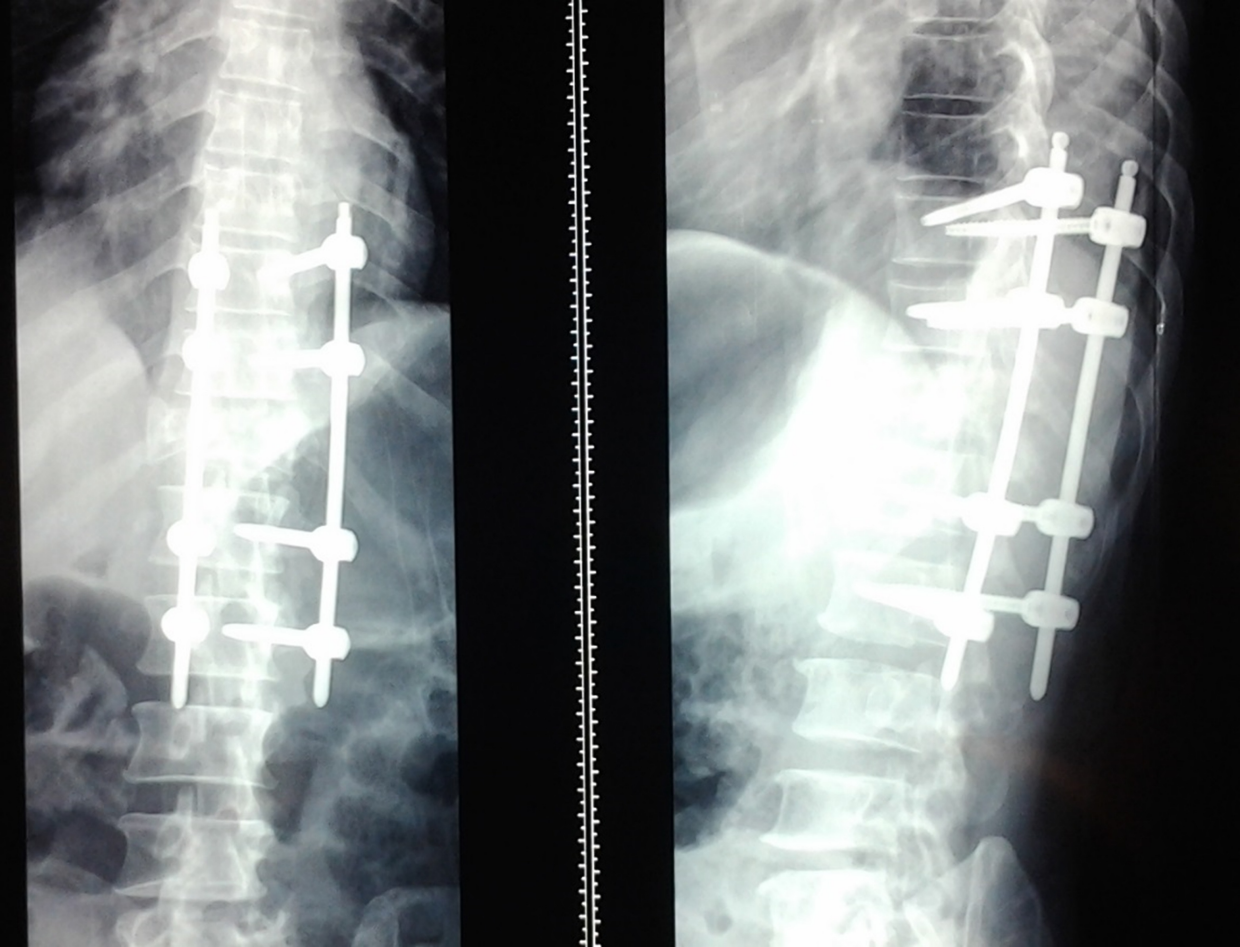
Postoperative Follow-up AP (left) and lateral (right) x-ray taken two weeks postoperatively demonstrating bridging instrumentation.
